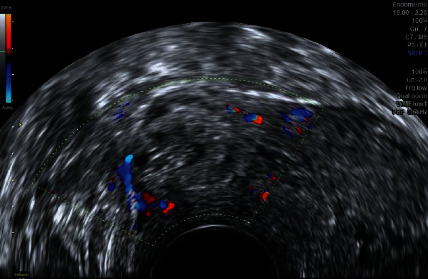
The color Doppler study showed vascularization.
